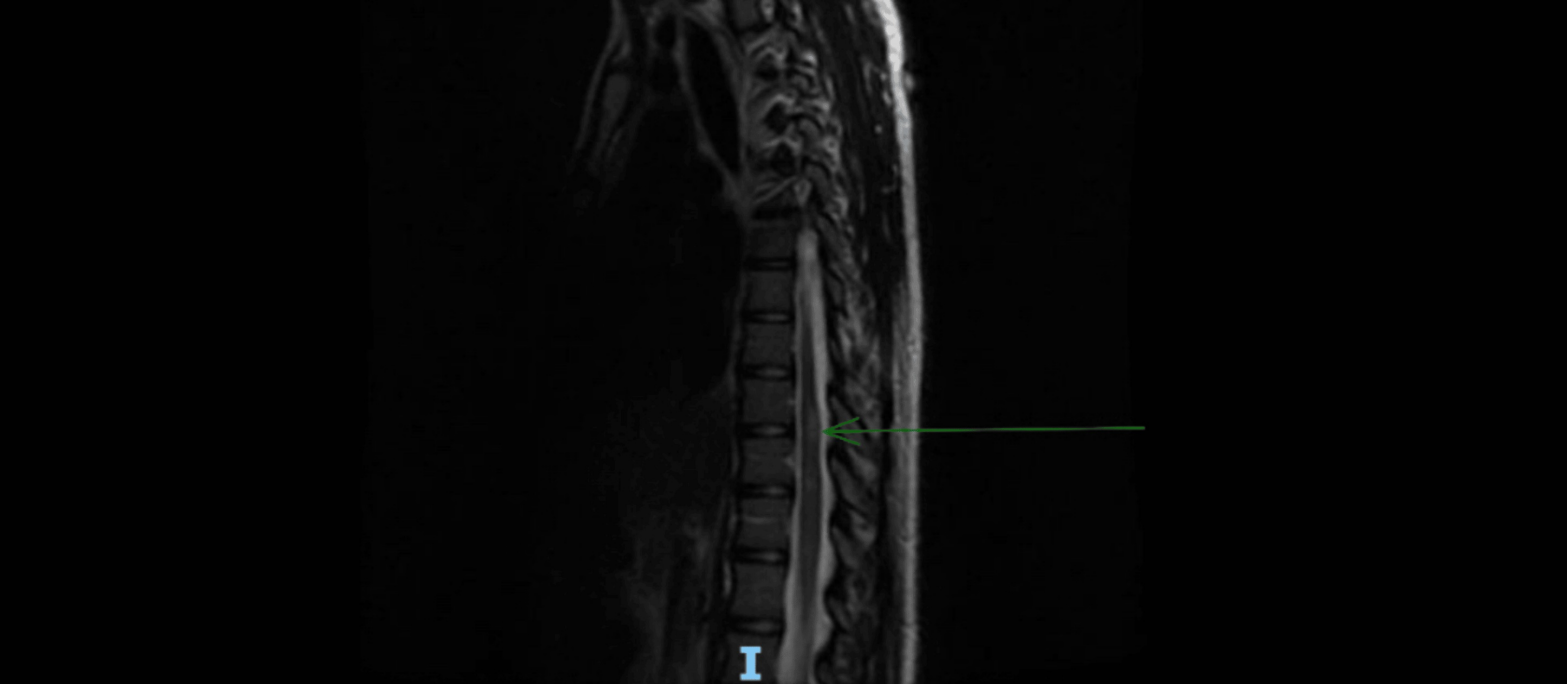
MRI of the thoracic spine T2 fast-recovery fast spin-echo sequence (sagittal view) showing T10-T12 hyperintensities MRI: magnetic resonance imaging
